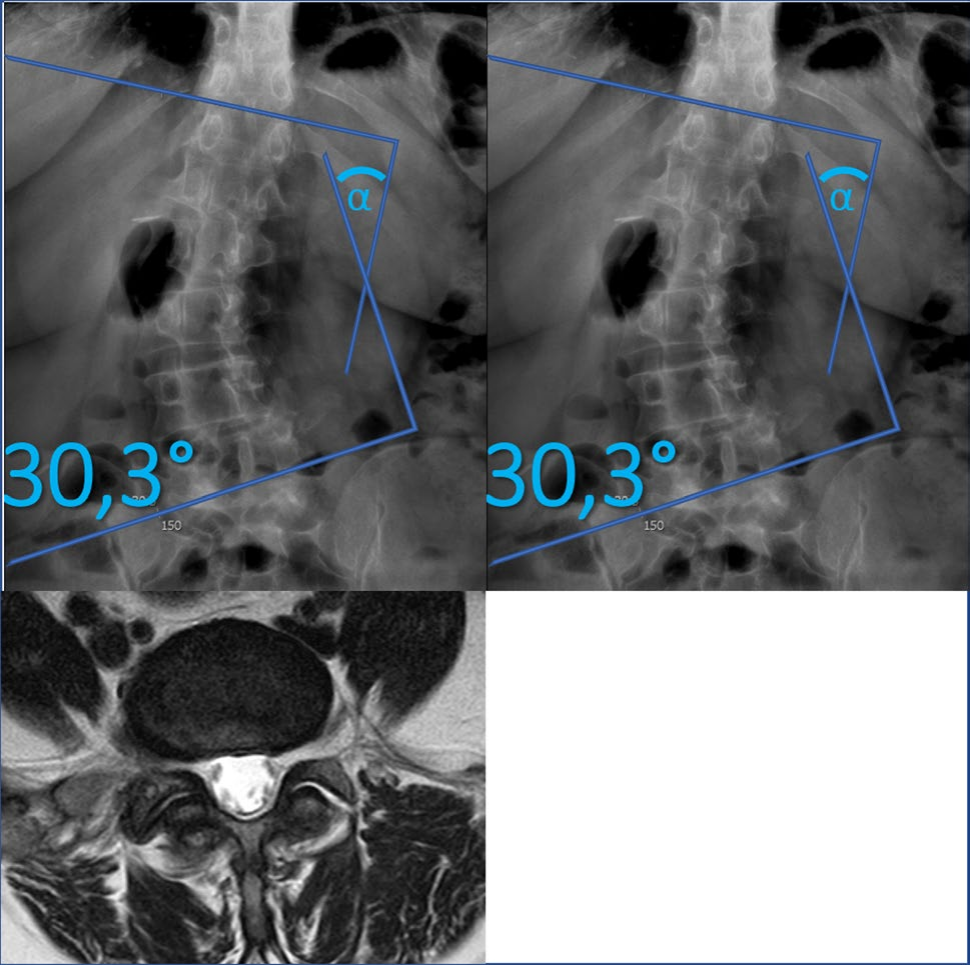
Moderate scoliosis with a Cobb angle of 30.3° plus signs of severe LFA due to marginal osteophytes, sclerosis hypertrophy, and a cyst on the right facet (AP X-ray, sagittal and axial T2-wighted MRI).
